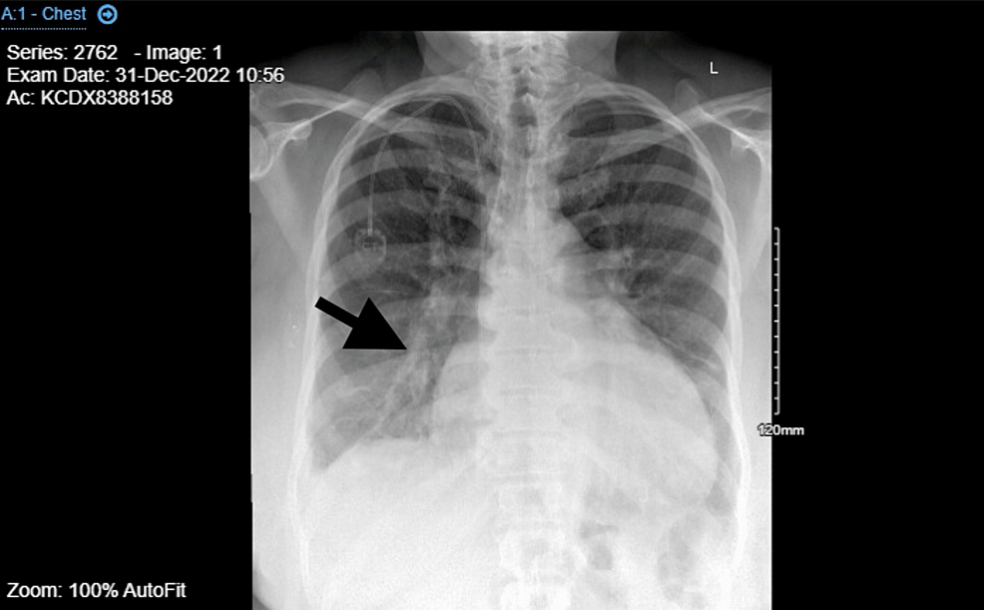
Chest X-ray on 12/5/2023 revealing severe pulmonary edema
